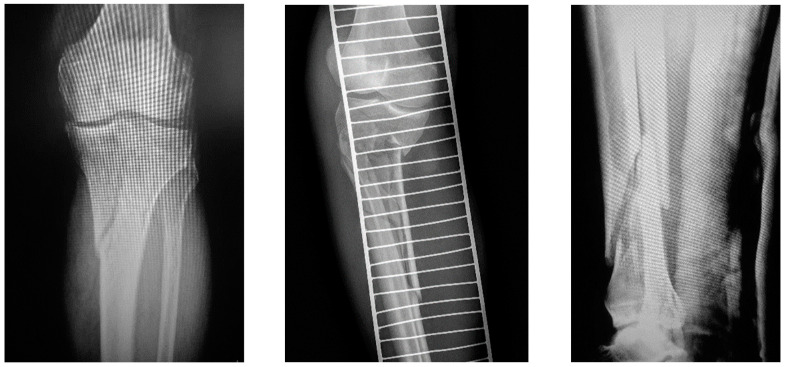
Preoperative radiographs of patients with an AO/OTA 41-C2.2 proximal tibial fracture with simple intra-articular involvement of both tibial condyles without intra-articular comminution and impaction (left), a 41-A3.1 multi-fragmentary proximal tibial extra-articular fracture (middle), or a 43-A2.3 distal tibial multi-fragmentary meta-diaphyseal fracture (right), all accompanied by significant soft tissue injury.
